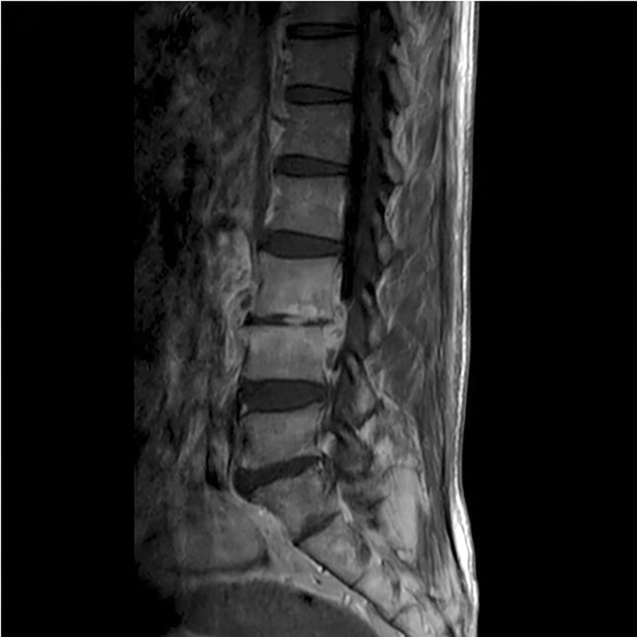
Magnetic resonance imaging showing bone destruction in the L3 and L4 vertebrae, narrowed intervertebral space, and paravertebral abscesses resembling teardrops.
